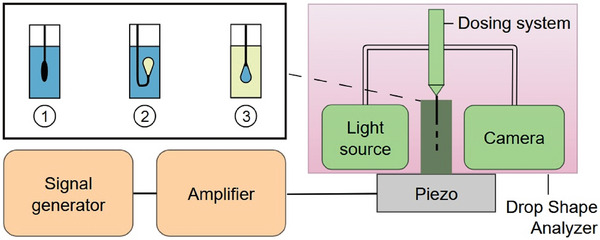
Depiction of the used setup for drop shape analysis under the influence of ultrasound. The piezo crystal was glued to a cuvette and was operated via a signal generator and an amplifier. 1) For the acoustic pressure measurements the dispensing tip was replaced with a hydrophone. For the interfacial tension measurements either 2) rising drop (of 1‐dodecene) or 3) pendant drop technique was used.
